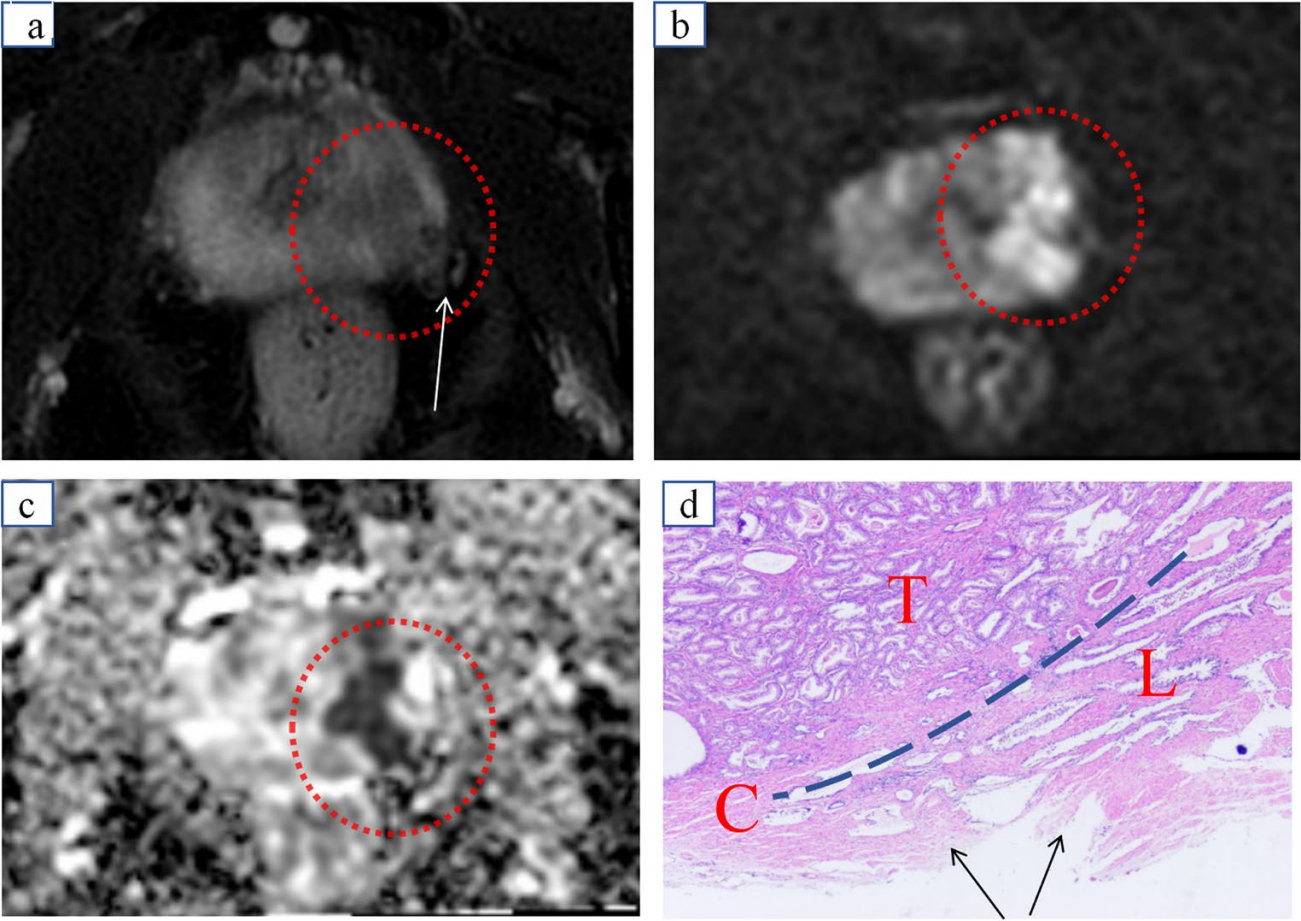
A typical case of false-positive misdiagnosis of EPE. The patient is a 72-year-old male. (a) T2WI shows a hypointense nodule in the left peripheral zone of the prostate (red circle) with a fibrous band shadow outside the prostatic capsule (white arrow); (b) DWI reveals a hyperintense signal (red circle); (c) ADC shows decreased values (red circle). The MRI diagnosis was prostate cancer. However, due to the interference of inflammation in the evaluation of the local prostatic capsule morphology, it was misjudged as EPE grade 1, leading to a diagnostic error. (d) Pathology: T represents the tumor, C and the blue curve indicate the prostatic capsule, the black arrow points to the pathological fibrotic focus, and L represents lymphocytes (HE *20)
